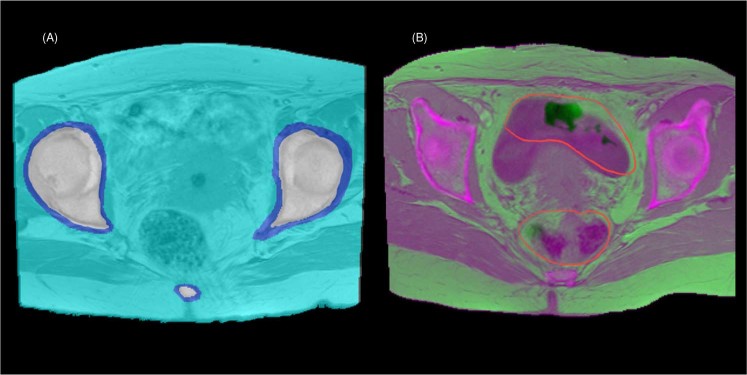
(A) Segmentation of the three regions superimposed on an MRI image: bony region (white), soft region (light blue), and mixed region (dark blue). (B) Example of a CT image (magenta) overlay on a corresponding MRI image (green) with the excluded region shown inside the red contours.
